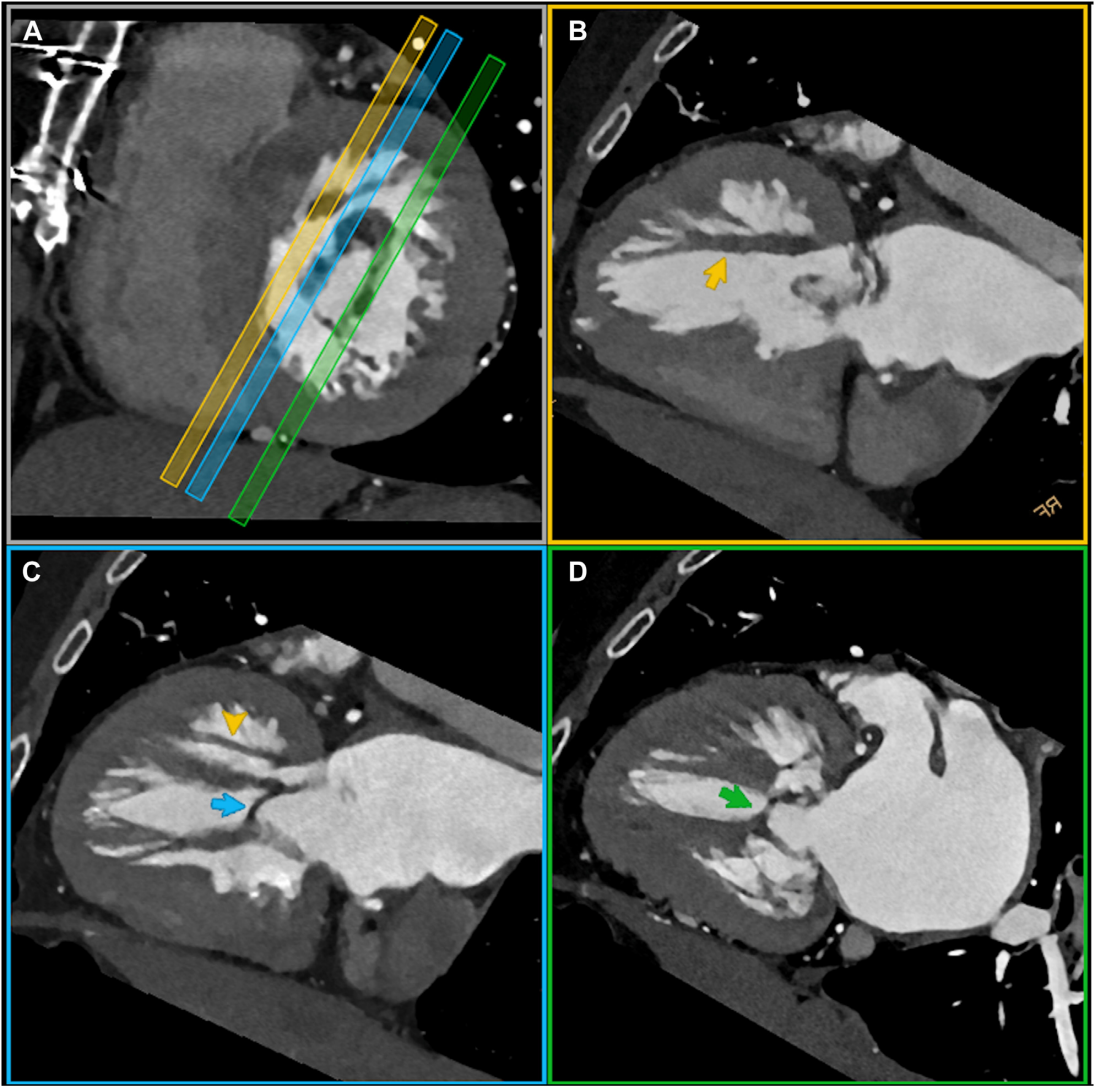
Multiplanar, basal short axis–guided (A), vertical long axis (B-D) cardiac computed tomography reconstructions through the pathologic mitral apparatus demonstrate the subvalvular orifice (1.4 cm^2^) defined anteriorly by the anterolateral papillary muscle, posteriorly by the tip of posteromedial papillary muscle, medially (*blue slice*), and laterally (*green slice*) by 2 fibrous bands (*blue and green arrows*). An accessory muscular bundle, parallel to the anterolateral papillary muscle and running from the apex to the anterior basal septum close to the mitral annulus, is best demonstrated in a more medially aligned reconstruction (*yellow slice*) and further highlighted in panels B (*yellow arrow*) and C (*yellow arrowhead*).
